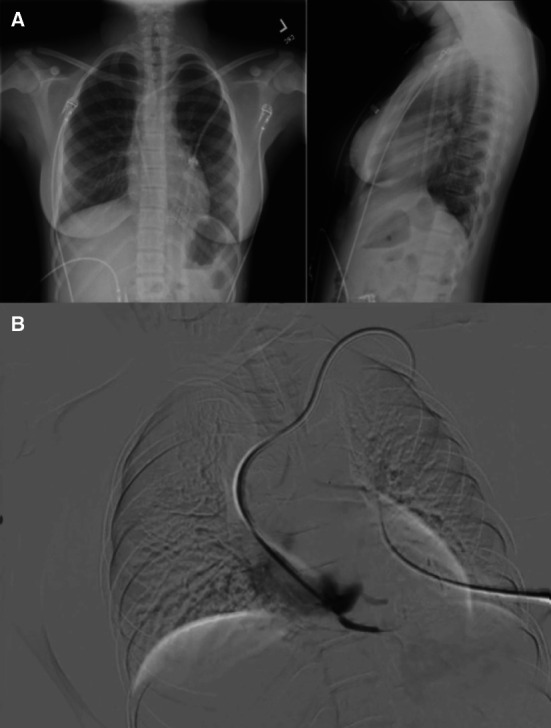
Chest x-rays and radiology-guided fluoroscopic study of hemodialysis catheter. (A) Anteroposterior and lateral chest x-rays showing the position of the tip of the left internal jugular vein catheter. (B) Interventional radiology–guided fluoroscopic study of the hemodialysis catheter showing contrast refluxing into the coronary vein (arrow).
